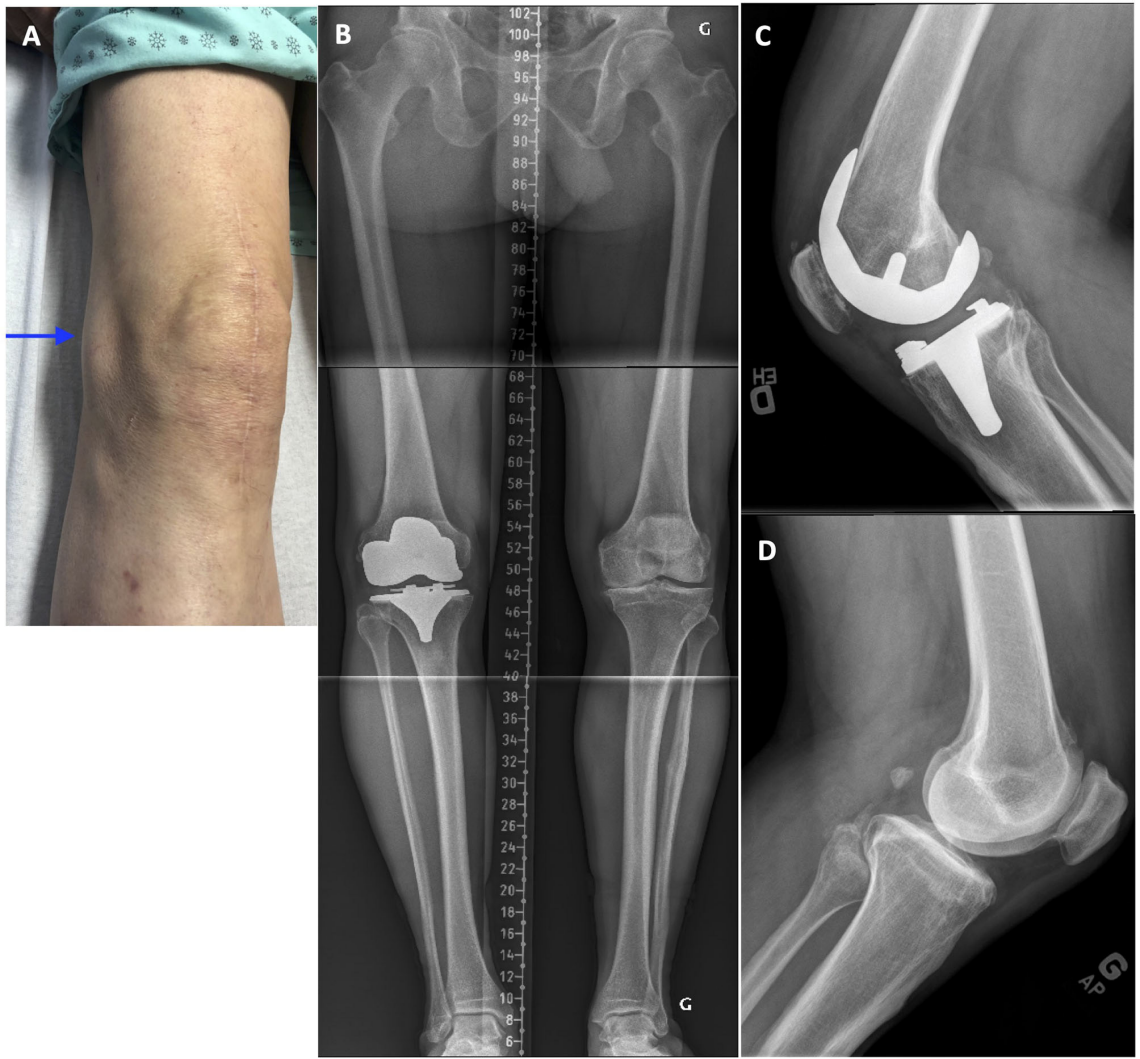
(A) Right knee clinical examination where swelling of the biceps tendon is observed. (B) Pre-revision long leg standing X-Ray reveals a right TKA implant with mDFA of 4° varus and a mPTA of 1.5° varus (5.5° varus aHKA). On the intact left side, the mDFA is 3.0° valgus and mPTA 3.5° varus (aHKA of 0.5° varus). (C) right knee lateral view where the implant posterior tibial slope is 6.5°. (D) Left knee lateral view where the native tibial slope is 2.0° posterior.
